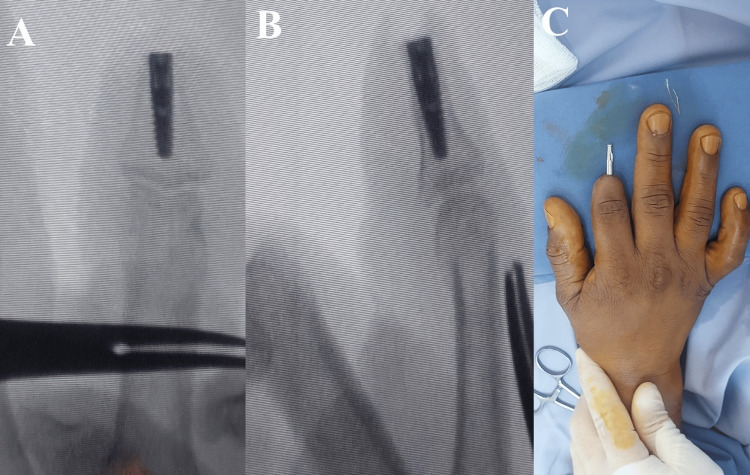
(A, B) Radiographic images demonstrating proper positioning of the dental implant within the middle phalanx. (C) Intraoperative image from the second-stage surgery showing fixation of the abutment to the dental implant.
